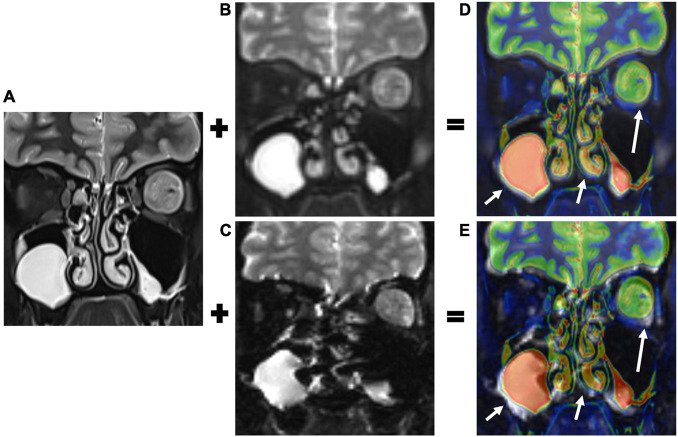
T2-weighted imaging (T2WI, A), b0 images of TGSE-PROPELLER-DWI (B), and rs-EPI (C) were fused. Color- and gray-coated images were derived from the T2WI and the two DWI b0 images (D,E), respectively. The T2WI and TGSE-PROPELLER-DWI b0 images matched well, showing the left orbital tumor (long arrows in D) and ethmoid and maxillary sinuses (short arrows in D). The T2WI and rs-EPI b0 images were mismatched due to geometric distortions in the corresponding structures (arrows in E).
